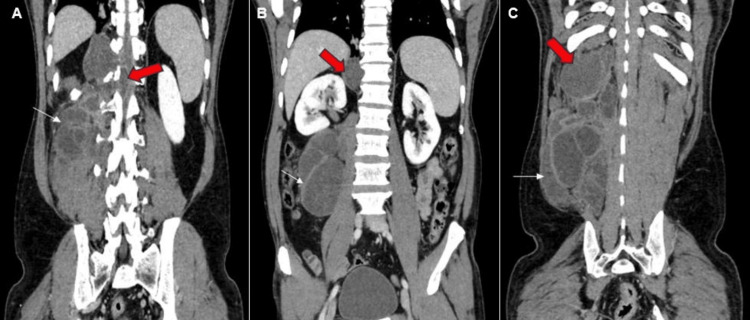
Reconstructed coronal sections of contrast enhanced computed tomography scan of the abdomen and the pelvis. (A) Intraspinal extension of the hydatid cyst (red block arrow). Multiple cysts are seen in the paraspinal muscles (white arrow). (B) Superior extension of the cysts displacing the right kidney (red block arrow). Posterior abdomen wall and paraspinal muscle showing multiple cysts (white arrow). (C) Superior extent of the lesion reaching the caudal surface of the diaphragm (red arrowhead) and multiple daughter cysts (white arrow).
